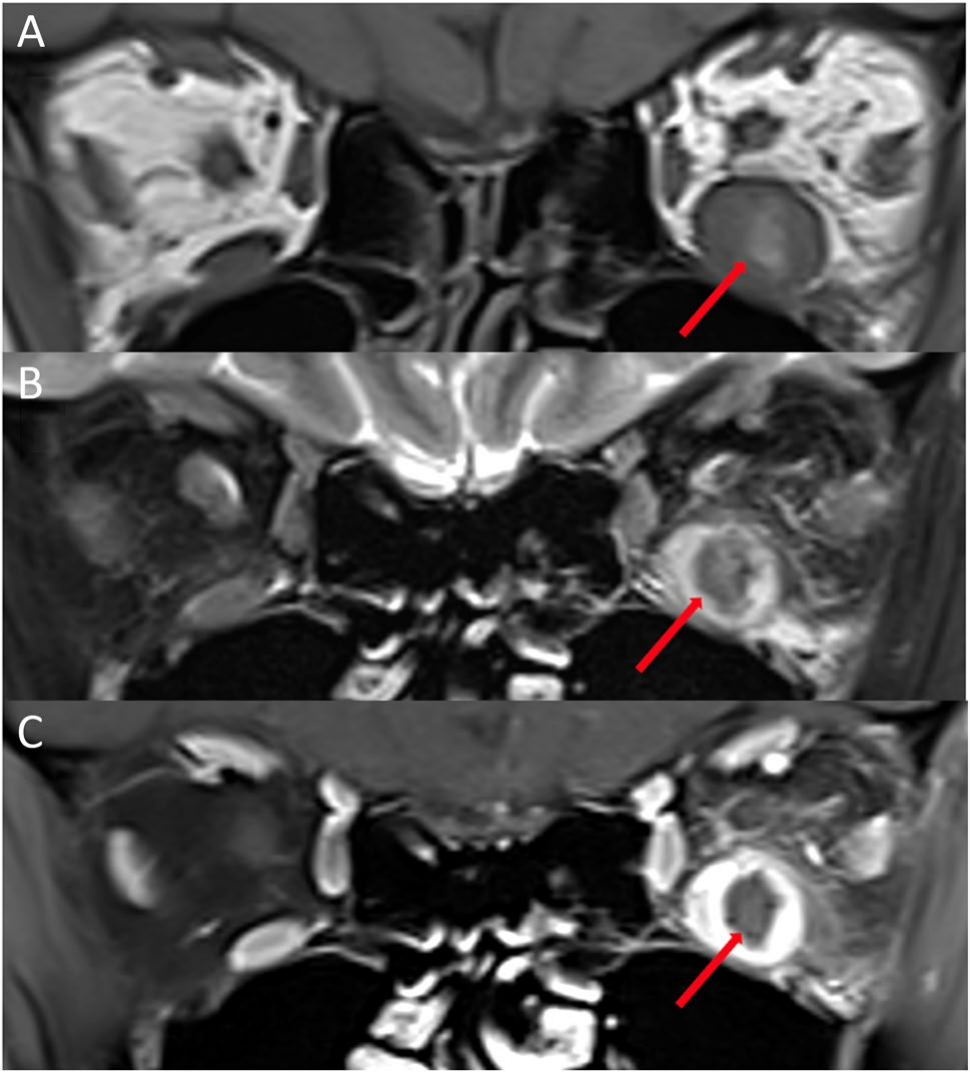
Neuroendocrine tumour metastasis. Coronal T1-weighted MRI (A), fat-suppressed T2-weighted MRI (B), and fat-suppressed contrast-enhanced T1-weighted MRI (C) showing enlargement of the left inferior rectus muscle with intralesional haemorrhage (arrows) suggested by the hyperintensity on T1-weighted scan (A) and hypointensity on fat-suppressed T2-weighted (B) and fat-suppressed T1-weighted contrast-enhanced scans (C)
